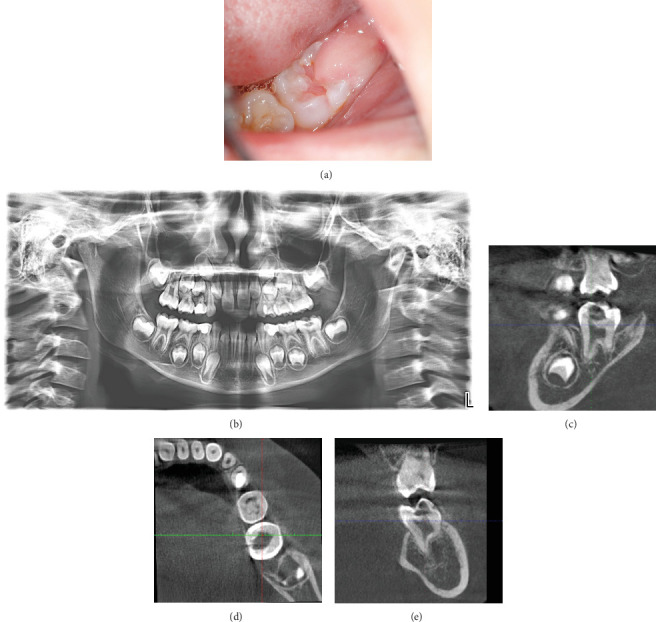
(a) Intraoral preoperative image; (b) panoramic X-ray taken 5 months earlier for orthodontic indications; (c) CBCT—sagittal view; (d) CBCT—transverse view; and (e) CBCT—coronal view.
